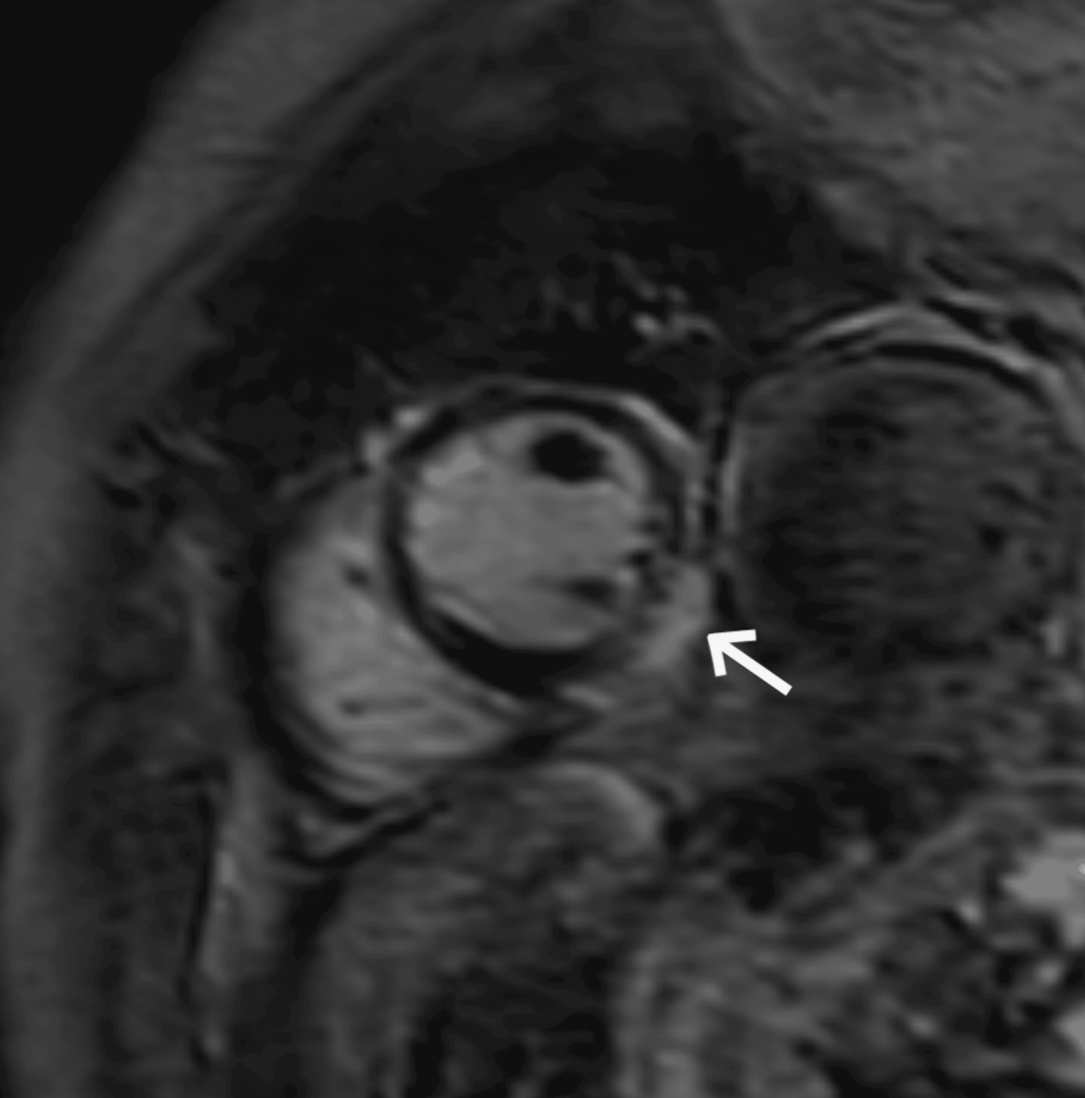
Delayed-enhanced MRI 10 minutes after gadolinium administration demonstrating sub-epicardial delayed enhancement (arrow) in the mid-inferolateral wall suggesting associated myocarditis
